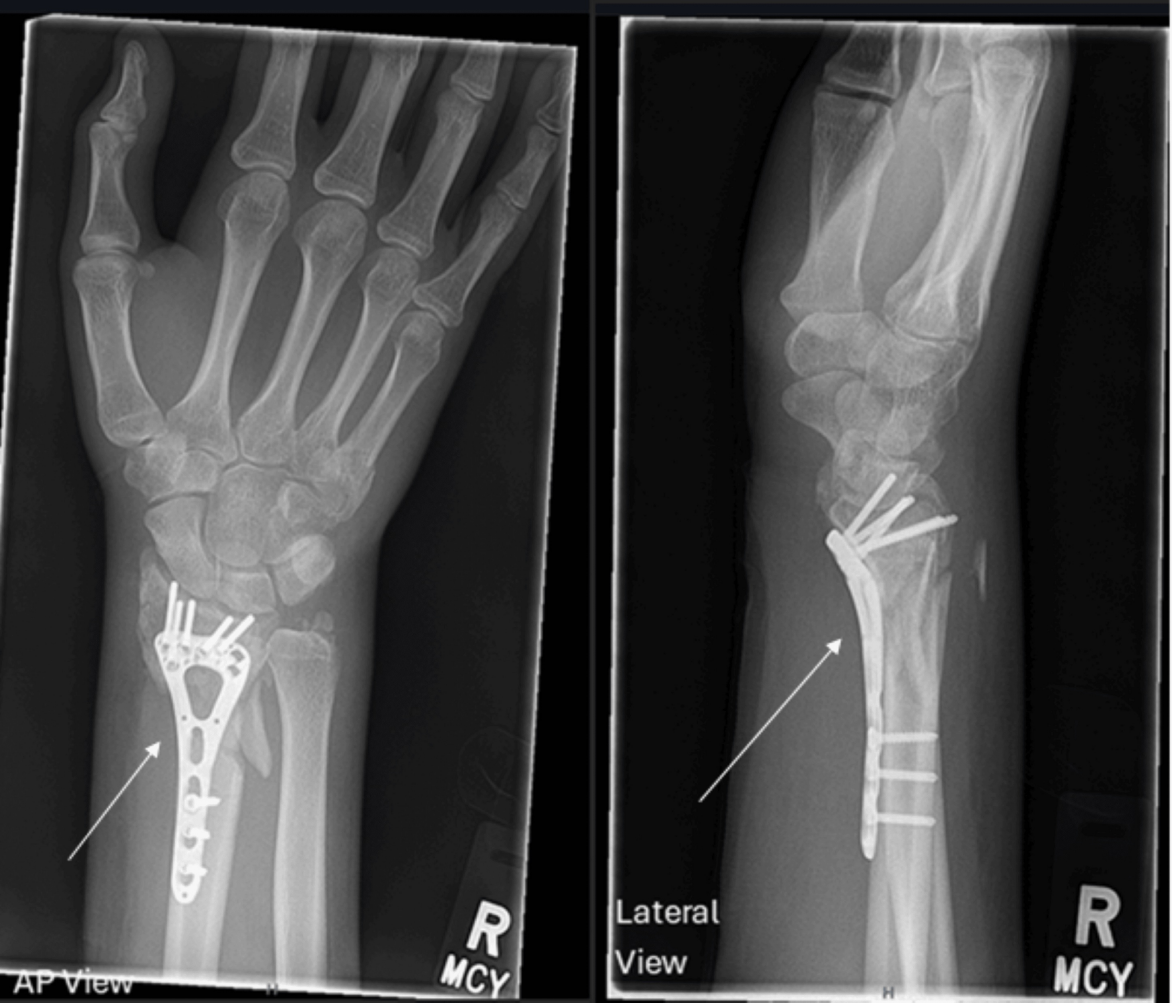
Postoperative X-rays following open reduction and internal fixation (ORIF) of the right distal radius with a volar locking plate (anteroposterior and lateral views). Arrows highlight plate position and fracture alignment.
